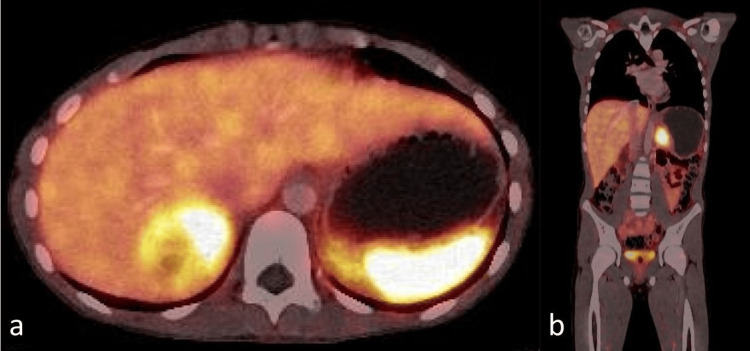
Whole-body ¹⁸F-aluminium fluoride (AlF)-NOTA-octreotide PET-CT revealed bilateral heterogeneous adrenal masses with markedly elevated radiotracer uptake, reflecting somatostatin receptor overexpression a: Right adrenal mass (5.5 cm; standardized uptake value (SUV)max 15.9); b: Left adrenal mass (3.2 cm; SUVmax 15.2), consistent with bilateral pheochromocytoma. There was no evidence of secondary dissemination.
